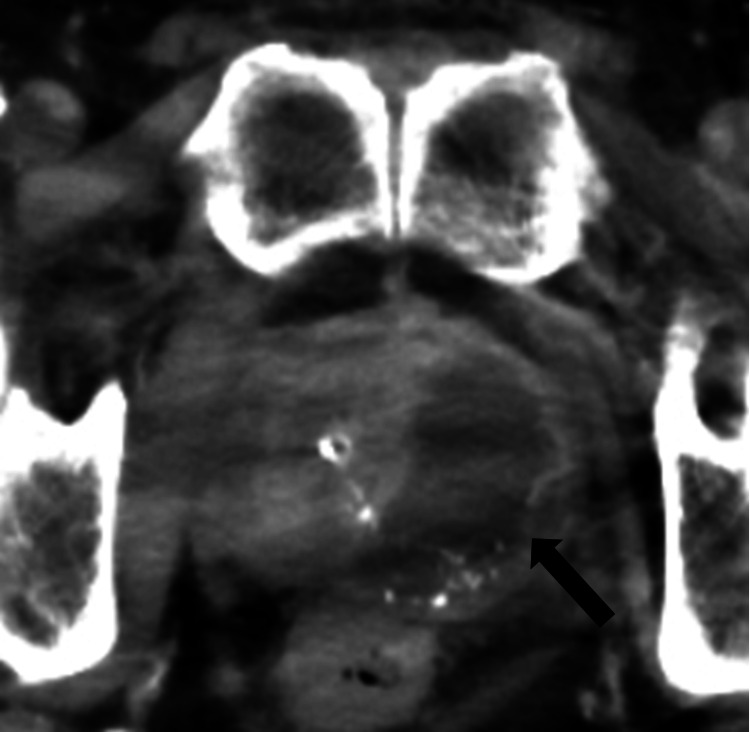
Pelvic non-contrast CT scan at the time of urinary retention A pelvic non-contrast CT scan at the time of urinary retention reveals a low-density area, 4.5 cm in diameter, located in the left lobe of the prostate (black arrow). The image was slightly blurred because of metal artifacts from the left total hip replacement. CT, computed tomography.
